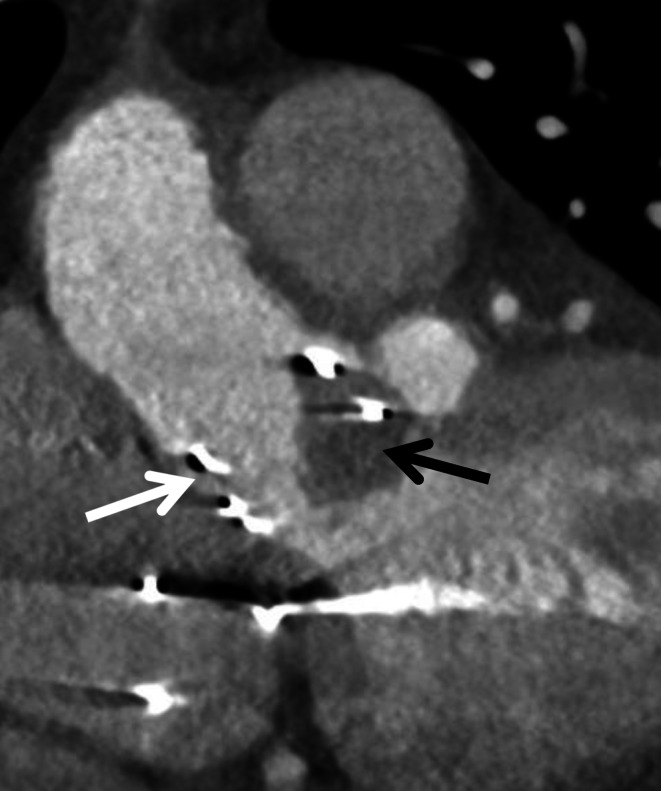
Image section of computed tomography in coronar view of the heart. Patient after previous transcatheter aortic valve implantation (TAVI) (*white arrow*) and acute thrombus detection on prosthesis valve struts (*black arrow*). This was regarded as the most likely cardiac embolic source responsible for acute ischemic stroke in this patient
